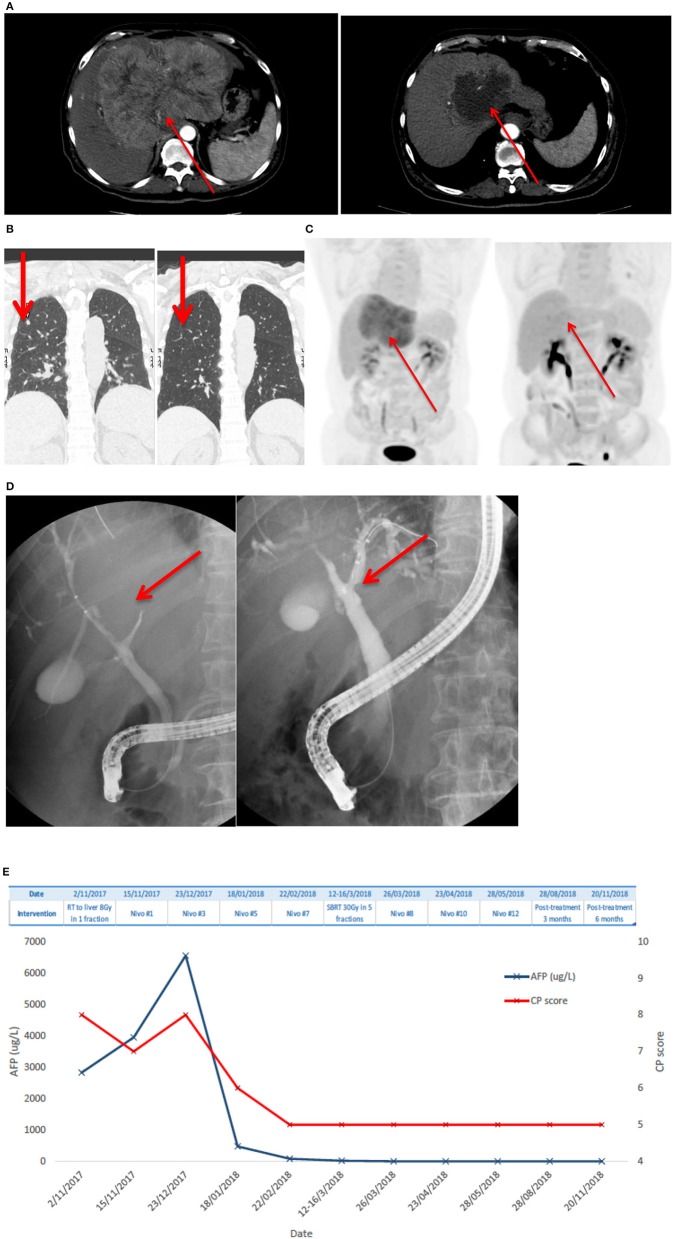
Patient #1 had advanced HCC and lung metastases who achieved complete remission after one single dose of 8 Gy SBRT and anti-PD1 antibody, followed by additional dose of 30 Gy in 5 fractions SBRT. (A) Shows a large arterial enhancing HCC over the left lobe of liver at diagnosis; there is marked reduction of tumor size and enhancing component of the lesion after radiotherapy and Nivolumab. (B) Demonstrates the complete resolution of lung metastases. (C) Shows that PET-CT revealed complete metabolic resolution of HCC. (D) Shows a compressed left main hepatic duct with minimally visible peripheral ducts on endoscopic retrograde cholangio-pancreatography (left) and resolved compression with fully visible ductal-branches after the treatment. (E) Shows AFP level and CP scores at different time points. AFP level improved and CP score improved over time.
